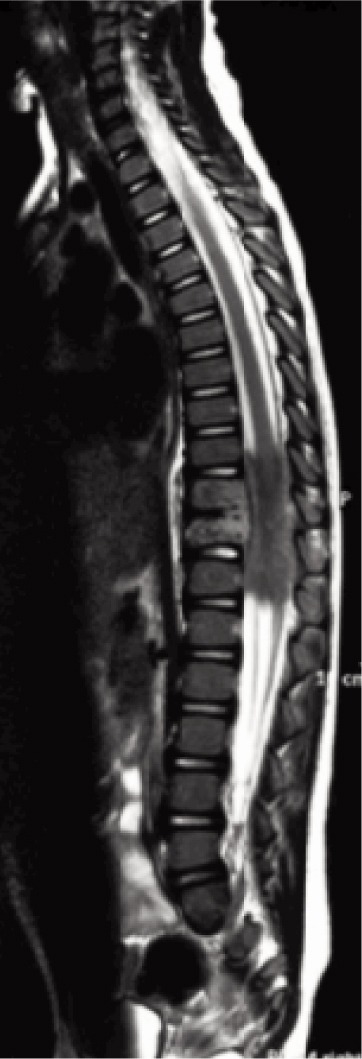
T2 weighted whole spine MRI.
